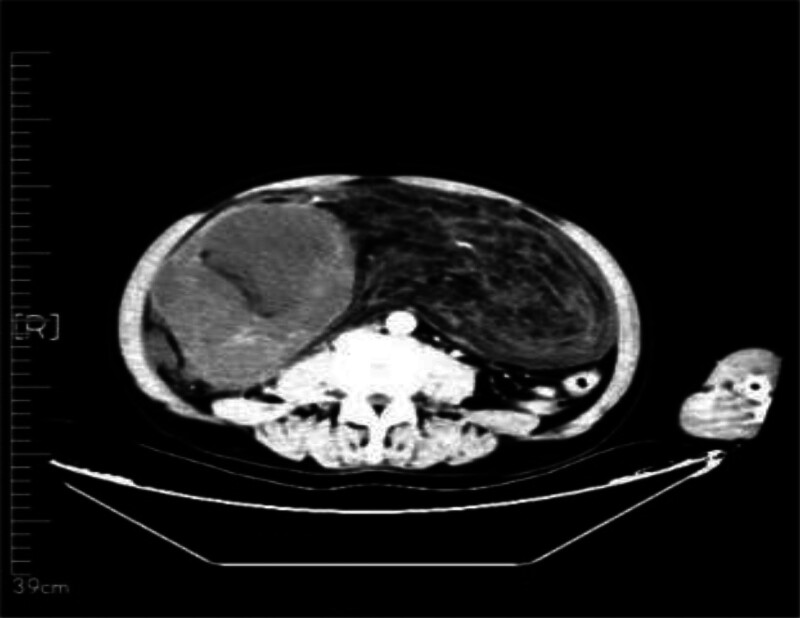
Obvious giant liposarcoma can be seen on CT.
